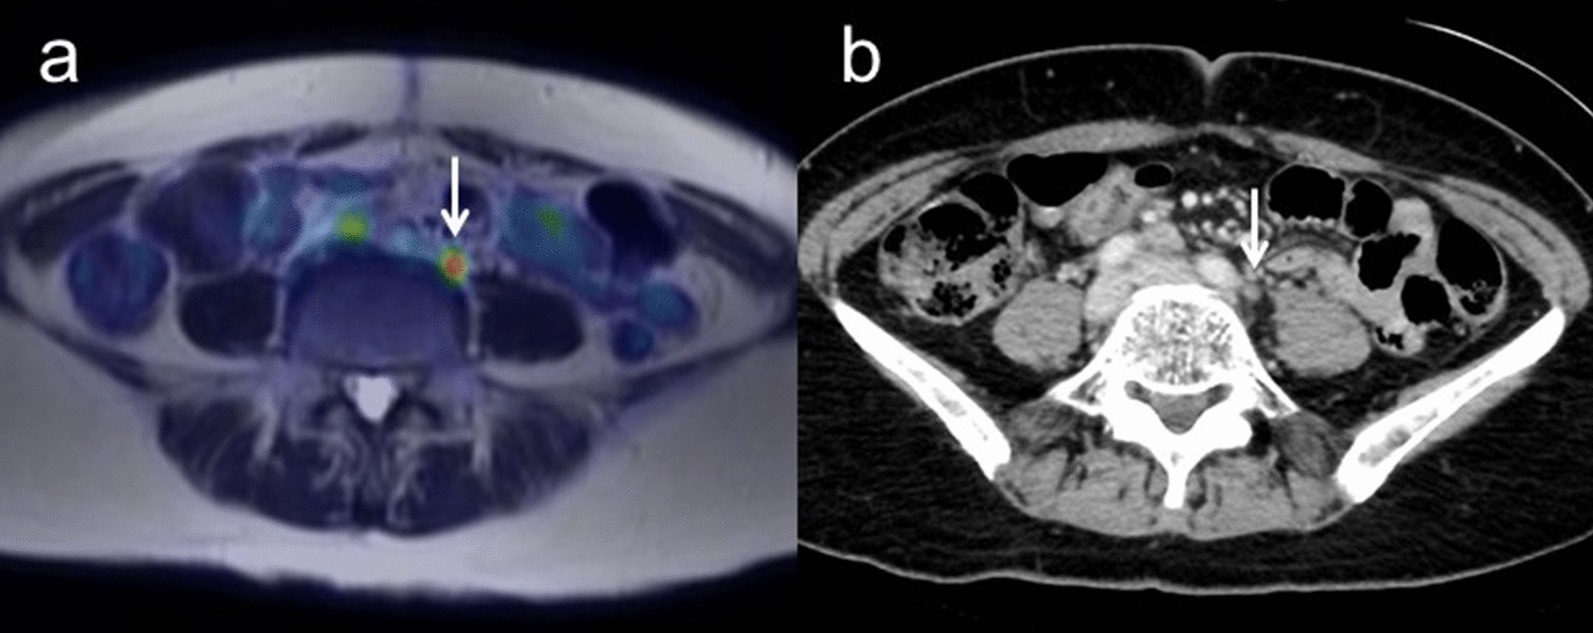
a A 63-year-old woman with pathologically confirmed ovarian cancer. During follow-up after the successful initial treatment, including surgery and first-line chemotherapy, CA-125 gradually increased. Axial T2-weighted PET/MR image shows the left para-aortic lymph node with FDG uptake (arrow). b Contrast-enhanced CT shows the left para-aortic lymph node less than 1 cm in short-axis diameter with slightly enhancement (arrow). After second-line chemotherapy, this lymph node decreased in size and SUV, suggesting this node as a sign of recurrence
